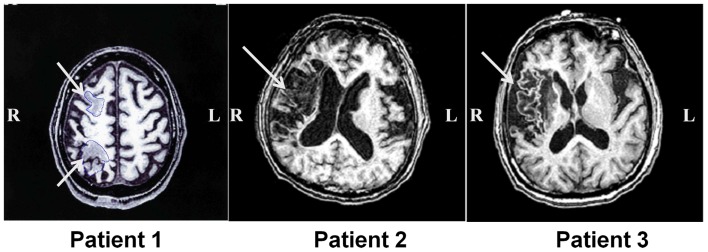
Lesion location in patients with unilateral spatial neglect. T1-weighted magnetic resonance images for all three patients with unilateral spatial neglect. White arrows indicate lesions.
